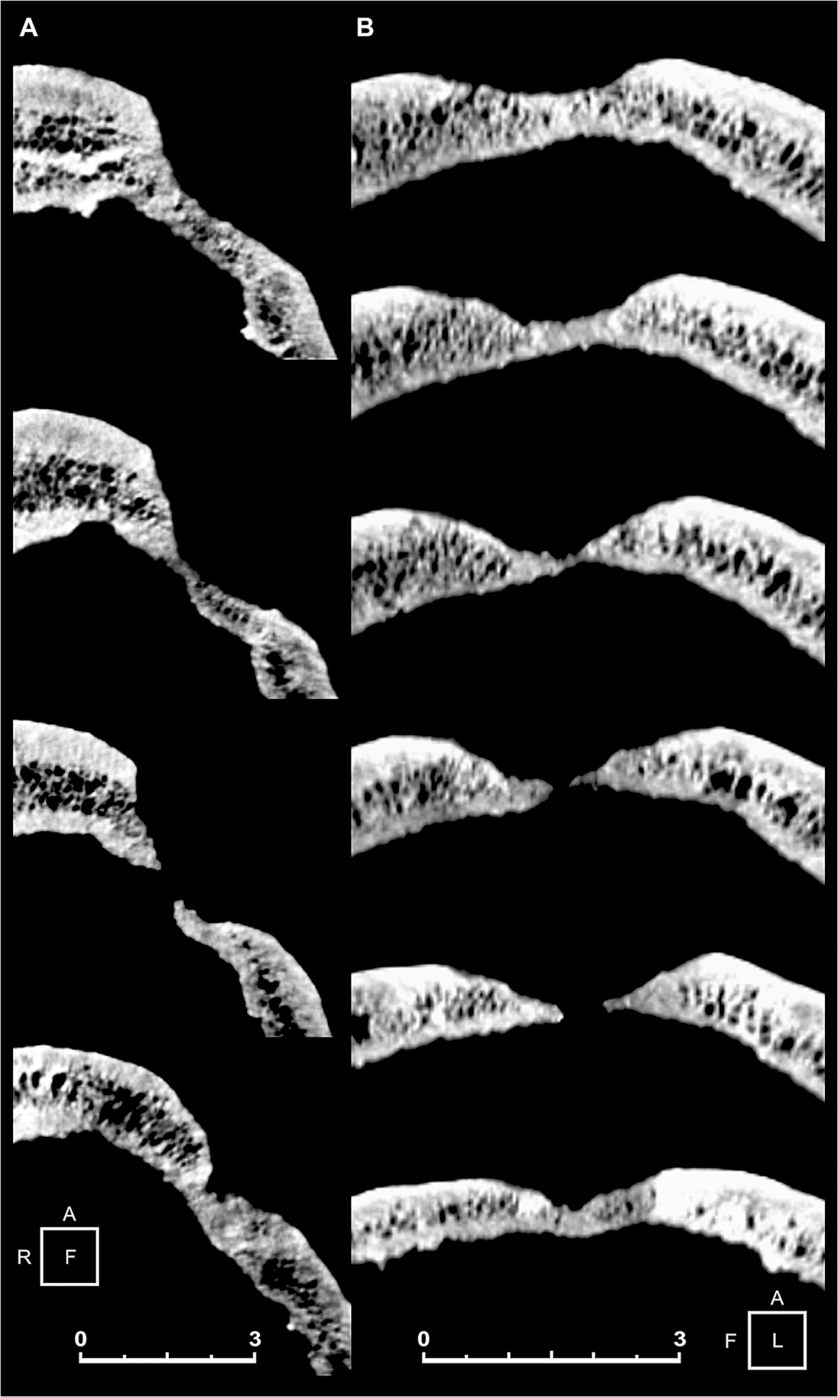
Three-dimensional (3D) computed tomography (CT) scan slices of the main skull trephination. Coronal (A) and sagittal (B) plane CT scan cross section sequence. Note the oblique orientation of the hole walls, and the defect edges remodeled into one compact bone layer, as a result of the loss of diploic structures. The smoothed, beveled edges are also indicative of bone regrowth. Scale bars measure 3 cm.
